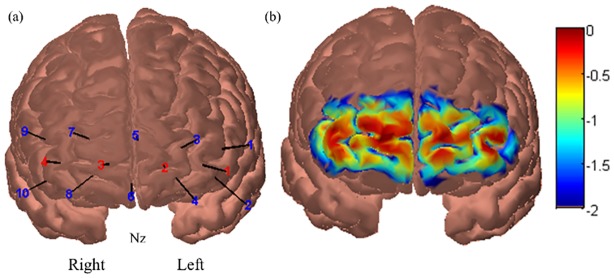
fNIRS probe placement. (a) Positioning of the 4 light sources (red) and 10 detectors (blue) with references to the nasion. (b) Sensitivity profile of the fNIRS probe used in this experiment projected onto a digital brain atlas based on the “Colin27” atlas [57] commonly used in MRI studies. The colour scale depicts the sensitivity logarithmically. Both images were created using AtlasViewerGUI [58].
